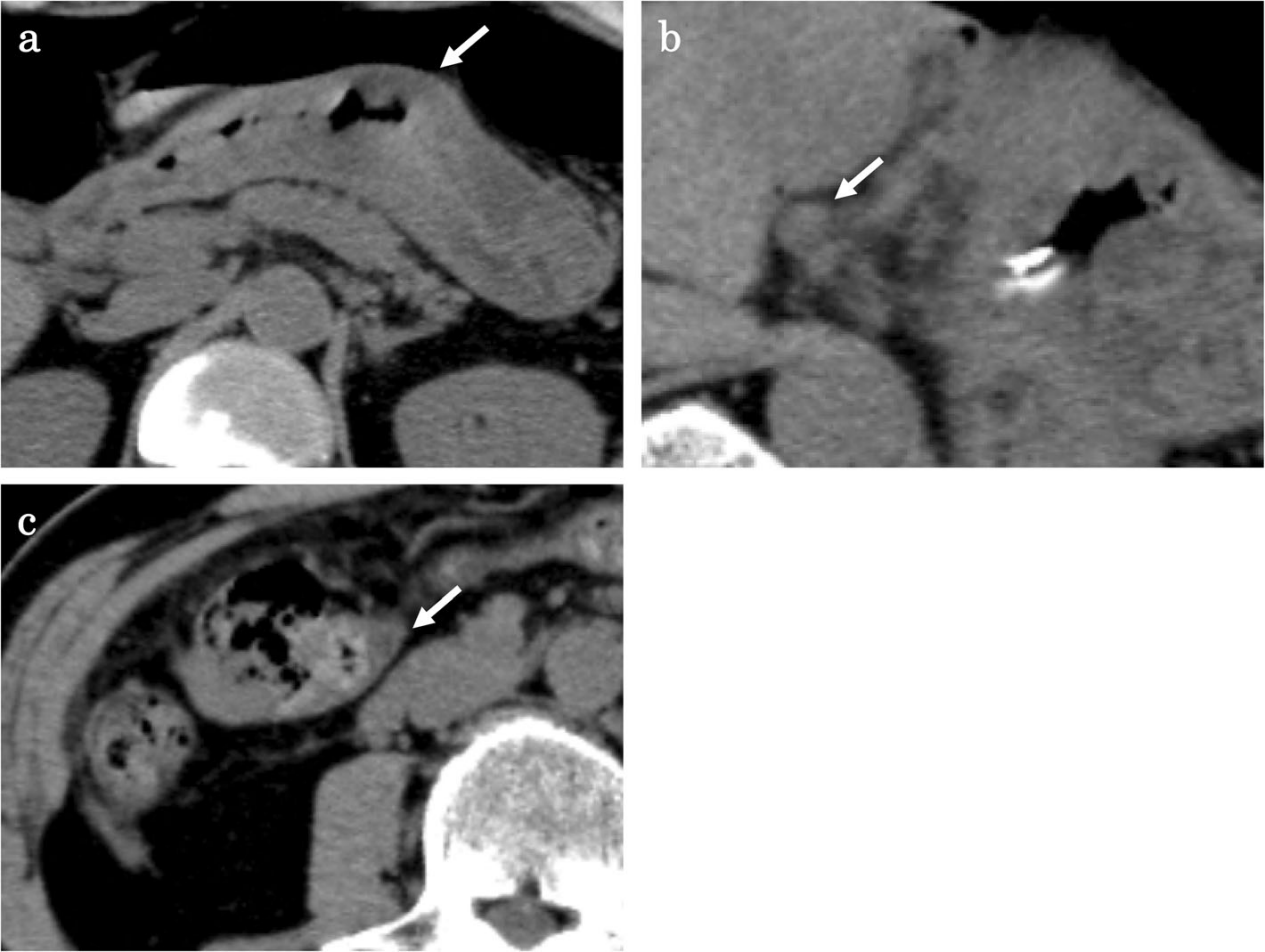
CT findings before chemotherapy. a CT scan showed irregular wall thickness at the gastric body (white arrow). b CT scan showed lymph node enlargement at station 3 with a size of 7 × 11 mm (white arrow). c CT scan showed irregular wall thickness in the transverse colon (white arrow)
